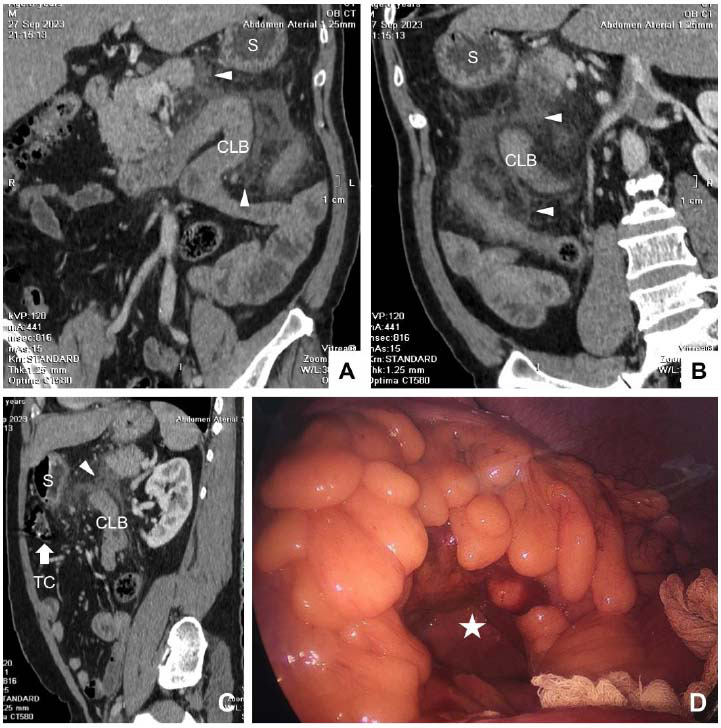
Multidetector-row computed tomography (MDCT) (A-C) and laparoscopic (D) images of a 53-year-old male patient experiencing dull epigastric pain. The MDCT images on the coronal (A) and oblique (B, C) planes showed a U-shaped loop of the small intestine, passing through the transverse colon (TC) mesentery near the angle of Treitz, that formed a hernia sac located behind the TC (white arrow), measuring 5.5 × 9.5 cm, with a thickened intestinal wall (9.3 mm), infiltration of adjacent fat tissue (arrowheads) in the hernia sac and at the root of the loop mesentery, suggesting localized enteritis, with no free fluid or air in the abdominal cavity. D: Laparoscopy of the TC mesentery showing an approximately 4 cm in diameter natural orifice (a white star) and 2 cm deep, with the first loop of the jejunum passed through it and signs of adhesion. CLB = Closed loop of bowel, S = Stomach, TC = Transverse colon.
